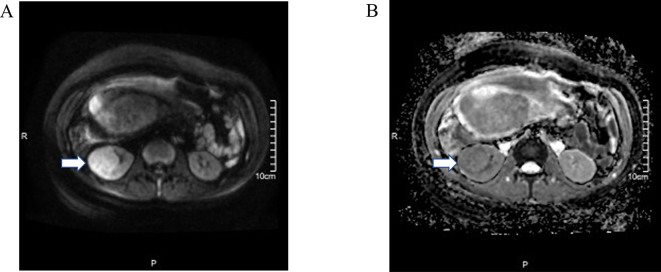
MRI demonstrated that the renal mass showed restricted diffusion, with DWI (A) showing hyperintensity and the ADC (B) revealing decreased values.
